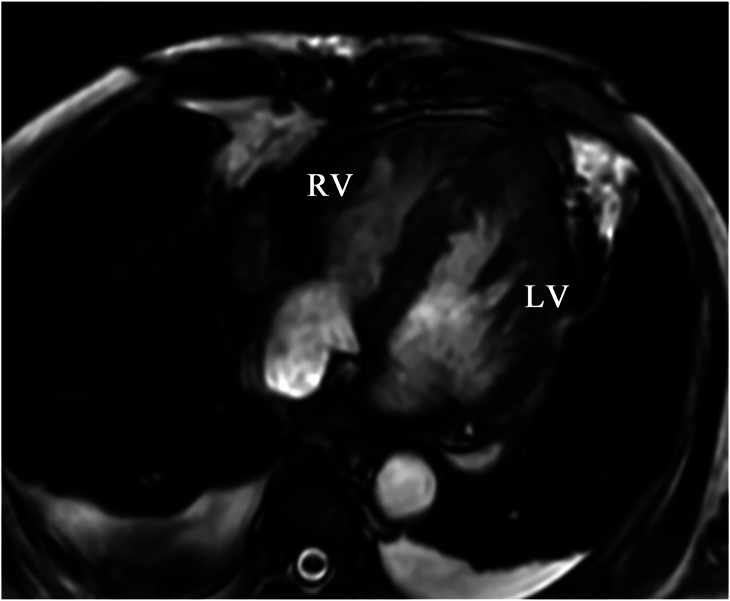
Nonenhanced magnetic resonance imaging of the heart shows no residual interventricular shunt. (LV, left ventricle; RV, right ventricle.)
